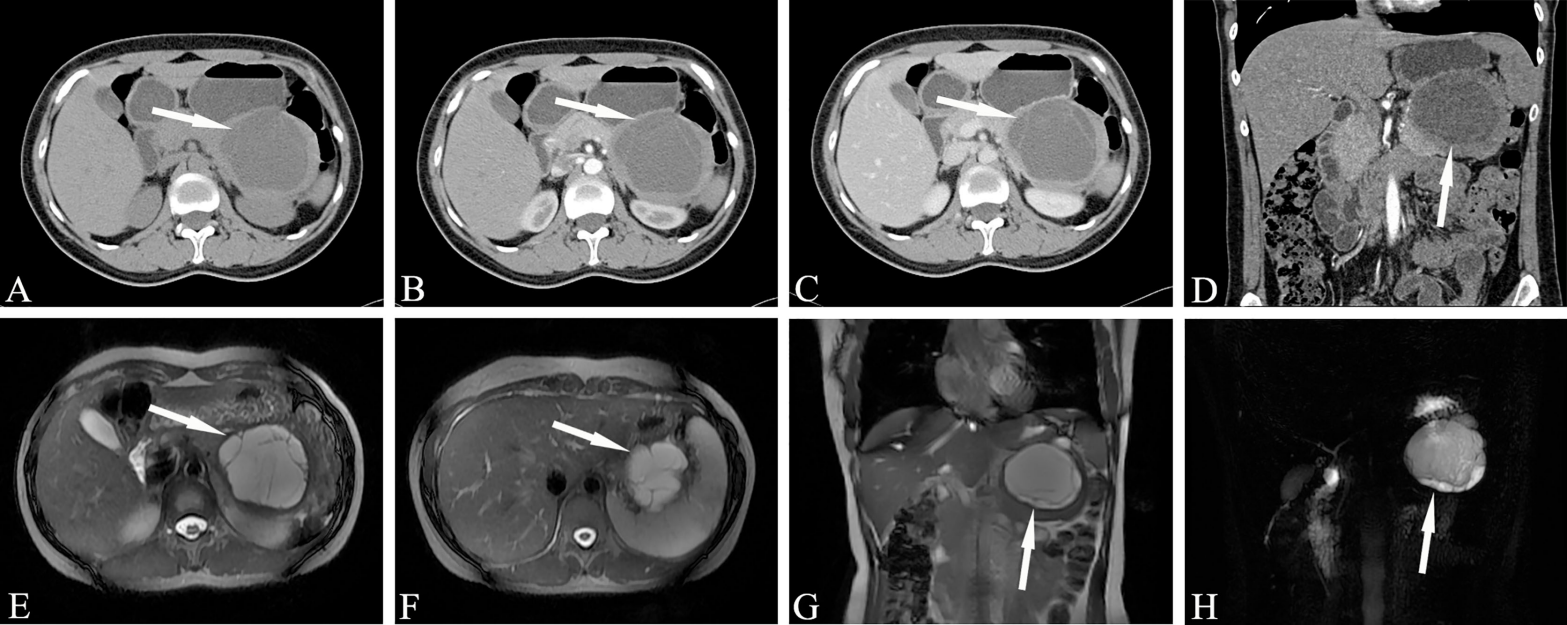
Mucinous cystic neoplasms, MCN (Female, 33y, physical examination revealed a pancreatic mass for one month). The sequence distribution of images is the same as that in Figure 2. (A–D) Huge cystic mass in the body and tail of the pancreas, irregular in shape, high tension, watery low density, thin and uniform cyst wall, multiple thin septum and small walled cysts can be seen in the cyst, and the septation and mild cyst wall can be seen enhanced after contrast injection. (E–H) Magnetic resonance images showed clearer septum and small sacs. There were no signs of pancreatic duct dilatation. The white arrows in the Figures only indicate the location of the neoplasm.
